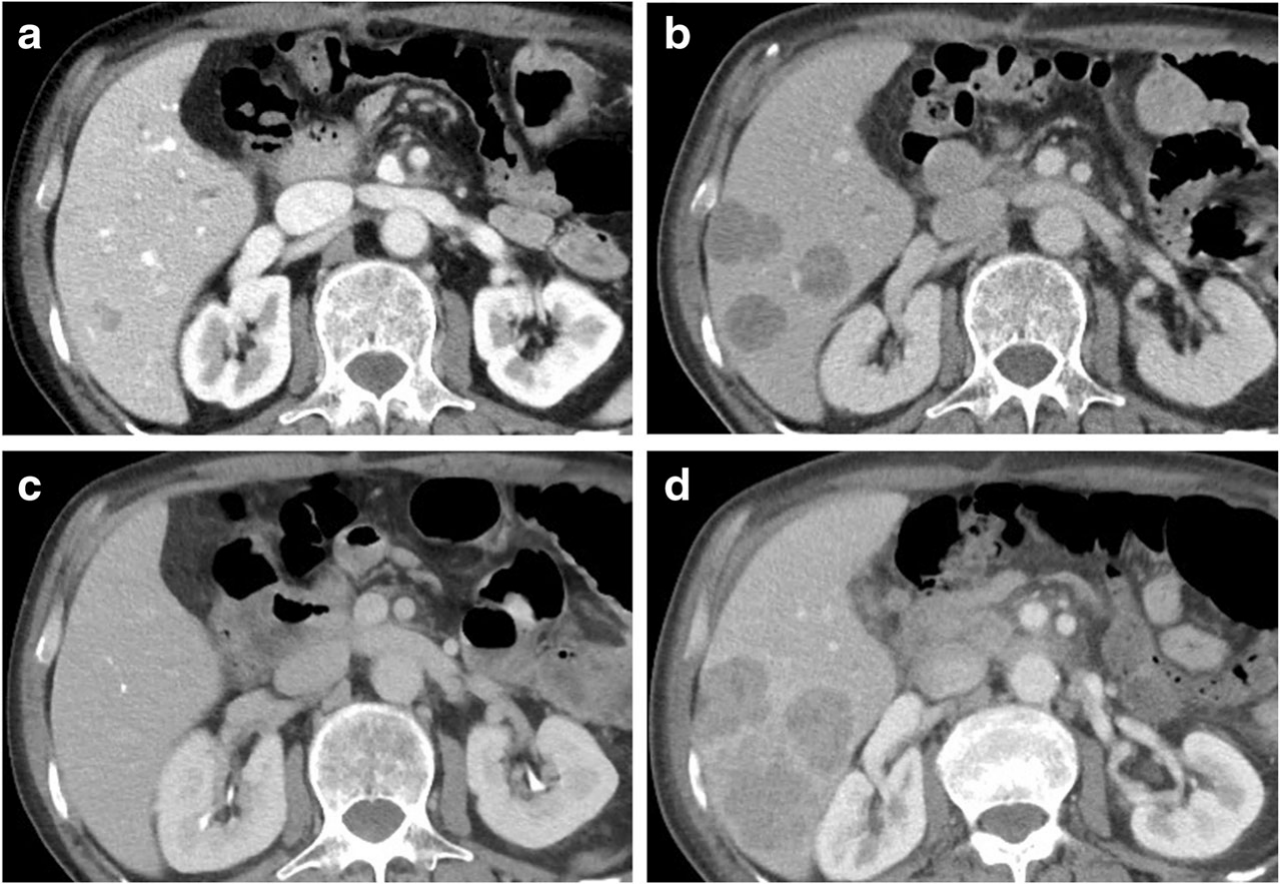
Serial abdominal computed tomography findings. Two months postoperatively, liver metastases are demonstrated (a), which increased 6 months postoperatively (b). The liver metastases resolved after 2 months of restarting gemcitabine plus cisplatin (c), but it enlarged again 2 months later (d)
